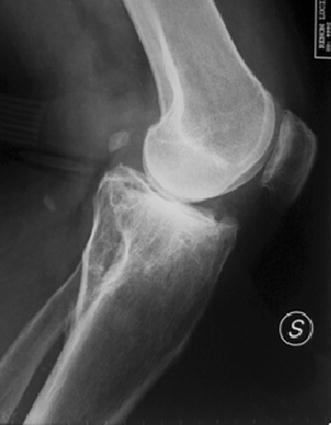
Schatzker type VI fracture, 1 year after X-rays (LL)
